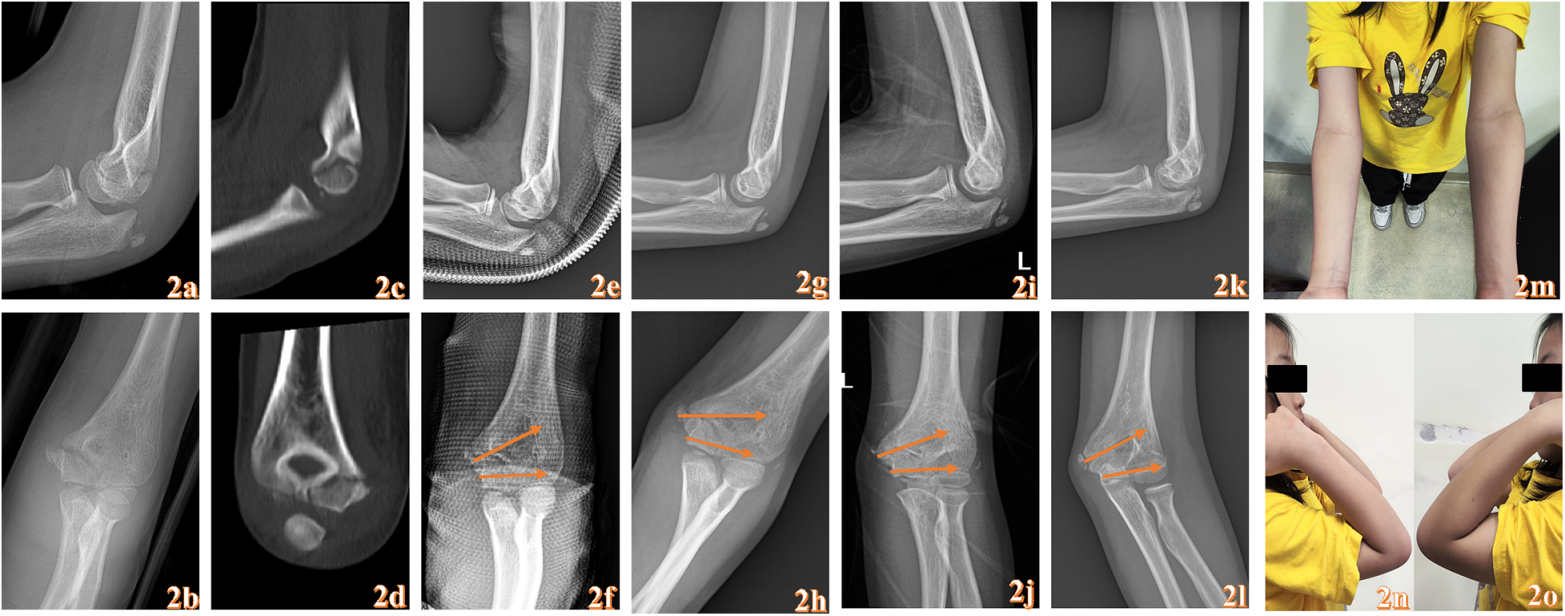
An 8-year-old female child who suffered pain in her left elbow due to a fall while running “pain with functional limitation 3d” admission. Anterolateral x-rays of the elbow joint before surgery (a,b). Preoperative CT (c,d). the anterolateral x-ray after operation (e,f). Postoperative anterolateral x-rays after 1 month (g,h). Postoperative anterolateral x-rays after 3 months (i,j). Postoperative anterolateral x-rays after 6 months (k,l). Appearance photographs taken 6 months postoperatively (m–o). The yellow arrow represents the placement of the absorbable cannulated screws.
